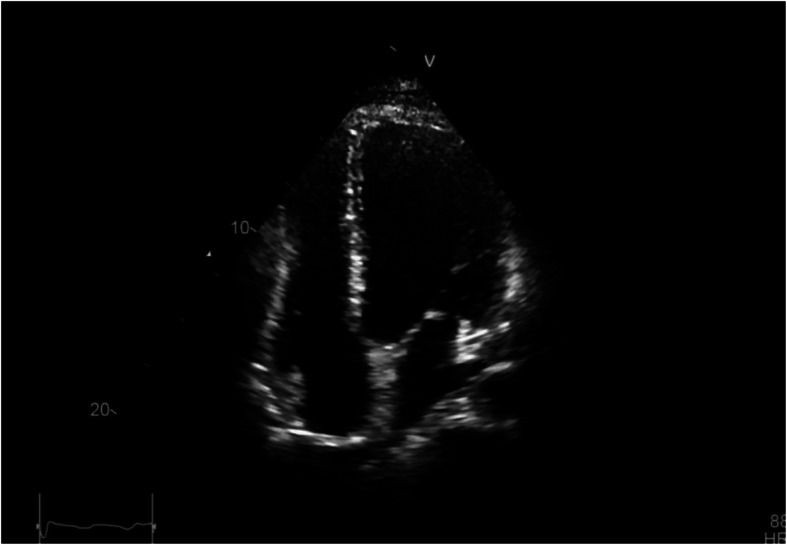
Exemplar of an apical four-chamber cardiac still image from the stimuli set
